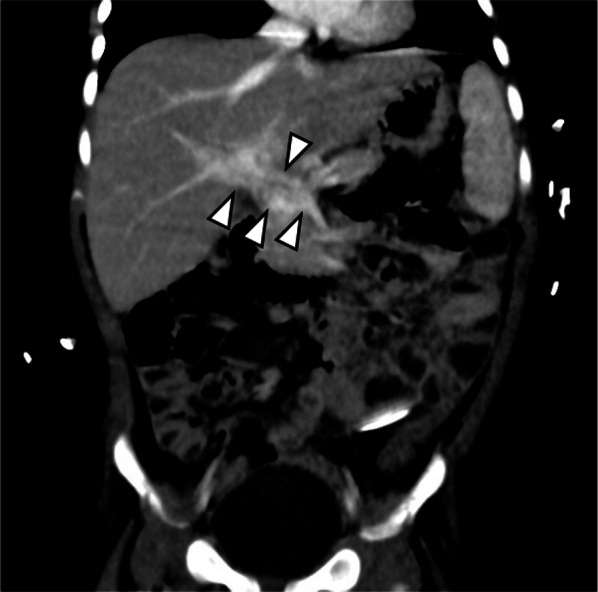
Contrast-enhanced computed tomography obtained at 87 days postoperative. Contrast-enhanced computed tomography (coronal reconstructed multiplanar image) revealed that a hypervascular tumor arising from the hepatoduodenal ligament was slightly reduced (arrowheads) after sirolimus (rapamycin) was started
